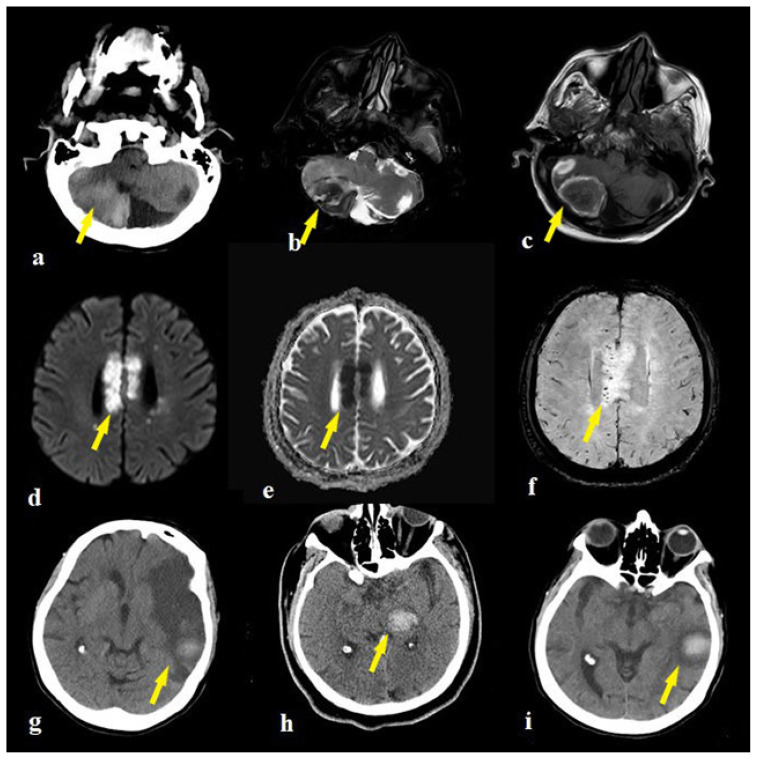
The right cerebellar hemorrhage appearences of a 74 years old man on CT (a) and axial T2W (b), T1W (c) MR images (arrows). The corpus callosum infarct of a 74 years old man on DWI/ADC (d,e) and microhemorrhages on SWI images (f) (arrows). The macrohemorrhages of a 64 years old female in the left MCA infarction zones (g,i) and a 46 years old man in the left thalamus (h) on CT (arrows).
